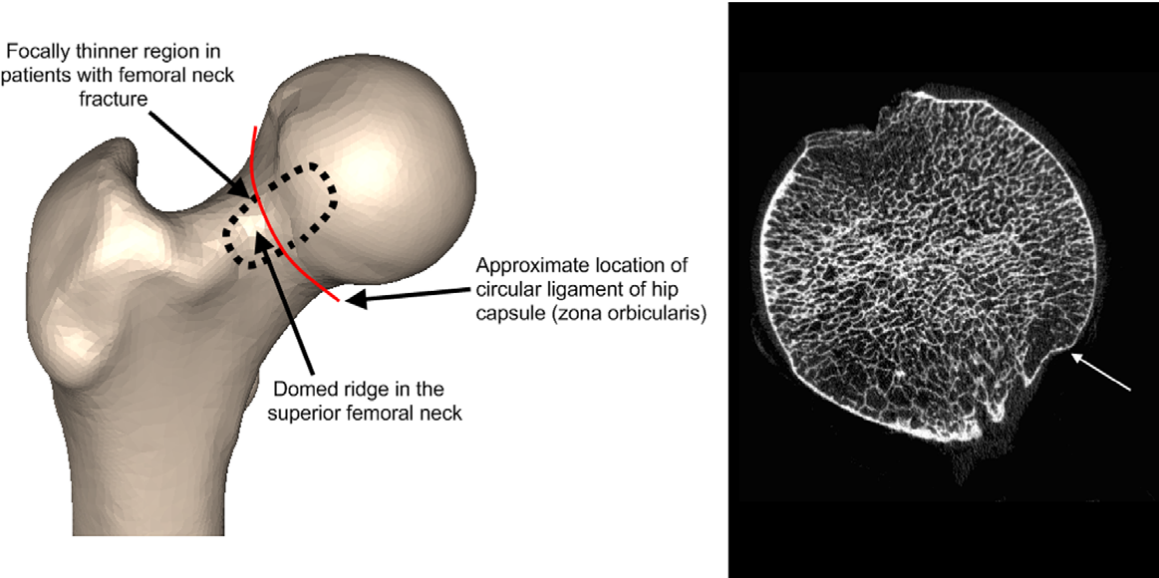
Anatomical context of focal thinning in women with femoral neck fracture. The left pane is a right proximal femur model seen from the front. The thin patch of cortex (fig 3c) in femoral neck fracture patients occurs on the domed ridge called the femoral neck eminence [25]. The right pane is a high resolution CT image through the femoral head of a 90 year old female (aBMD total hip T-score −1.9) which suggests that the patch is osteoporotic with microarchitectural thinning (white arrow). Femur courtesy of the Melbourne Femur Collection, Chairman Professor John Clement (Melbourne Dental School).
